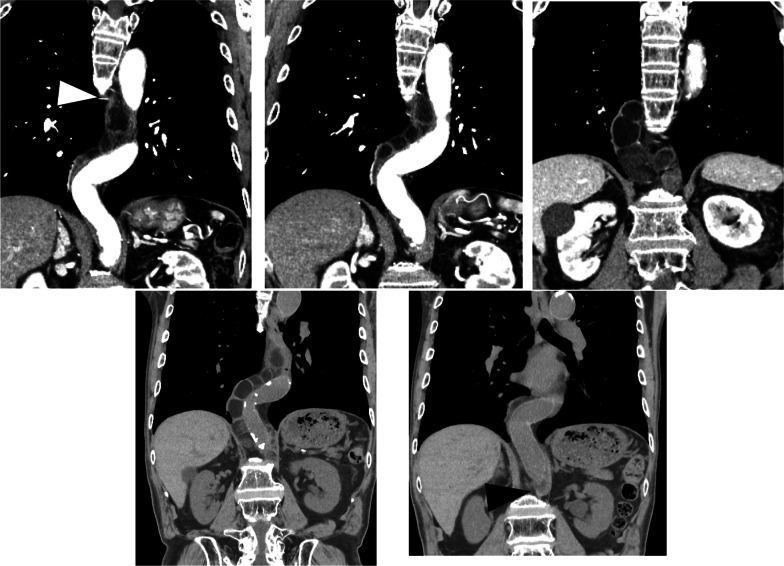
Computed tomography scan showing a continuous multifocal cystic lesion in the cephalocaudal direction from the posterior mediastinum to the retroperitoneum at the 8th thoracic to the 2nd lumbar levels. The white arrowhead (top left) indicates the most cephalad, and the black arrowhead (bottom right) indicates the most caudal
